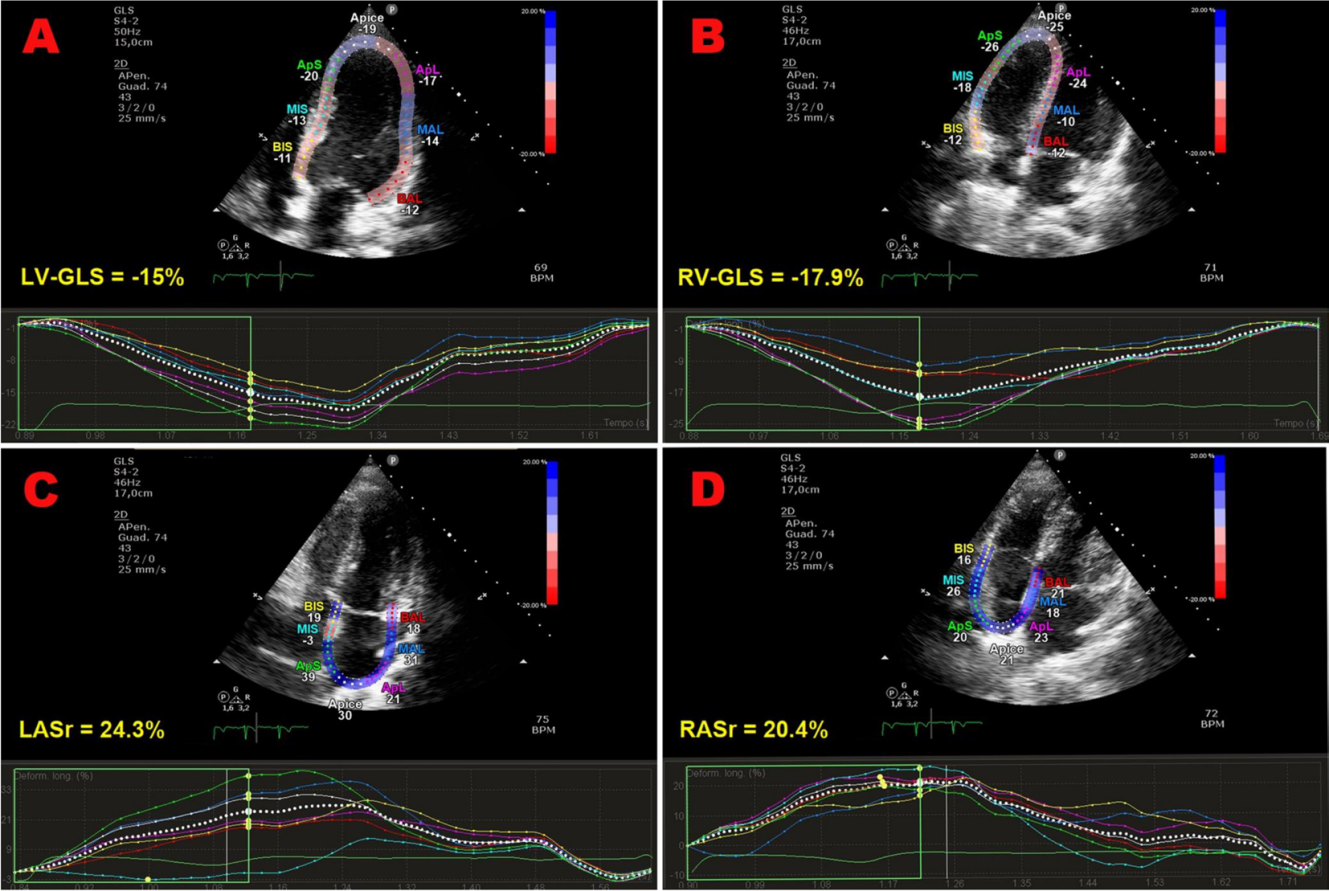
Representative examples of impaired biventricular and biatrial myocardial strain parameters obtained by strain echocardiographic imaging from the apical four-chamber view, in a ICI-treated cancer patient, over a short-term follow-up period. Absolute values of LV-GLS (A), RV-GLS (B), LASr (C) and RASr (D) were all moderately reduced in comparison to the accepted reference values. GLS, global longitudinal strain; ICI, Immune checkpoint inhibitors; LASr, left atrial reservoir strain; LV, left ventricular; RASr, right atrial reservoir strain; RV, right ventricular; STE, speckle tracking echocardiograp.
